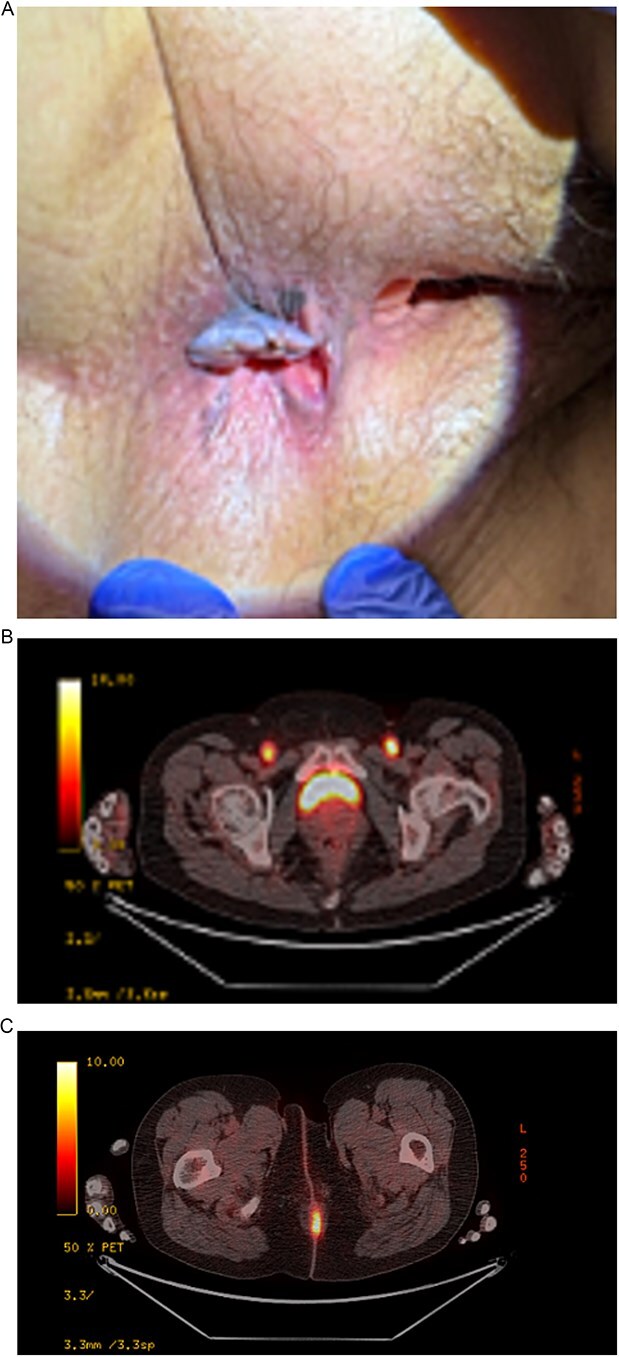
(A) 1 × 1 cm melanocytic mass in the right posterior peri-anal aspect. (B) PET/CT scan displaying bilateral FDG-avid inguinal lymphadenopathy with increased uptake. (C) Uptake in anal primary lesion.
